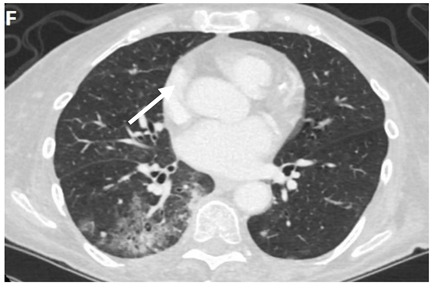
Axial CT chest (image F) showing predominantly ground-glass opacity and patchy focal consolidation in the RLL conforming to the area of radiation field denoted by an arrow.
